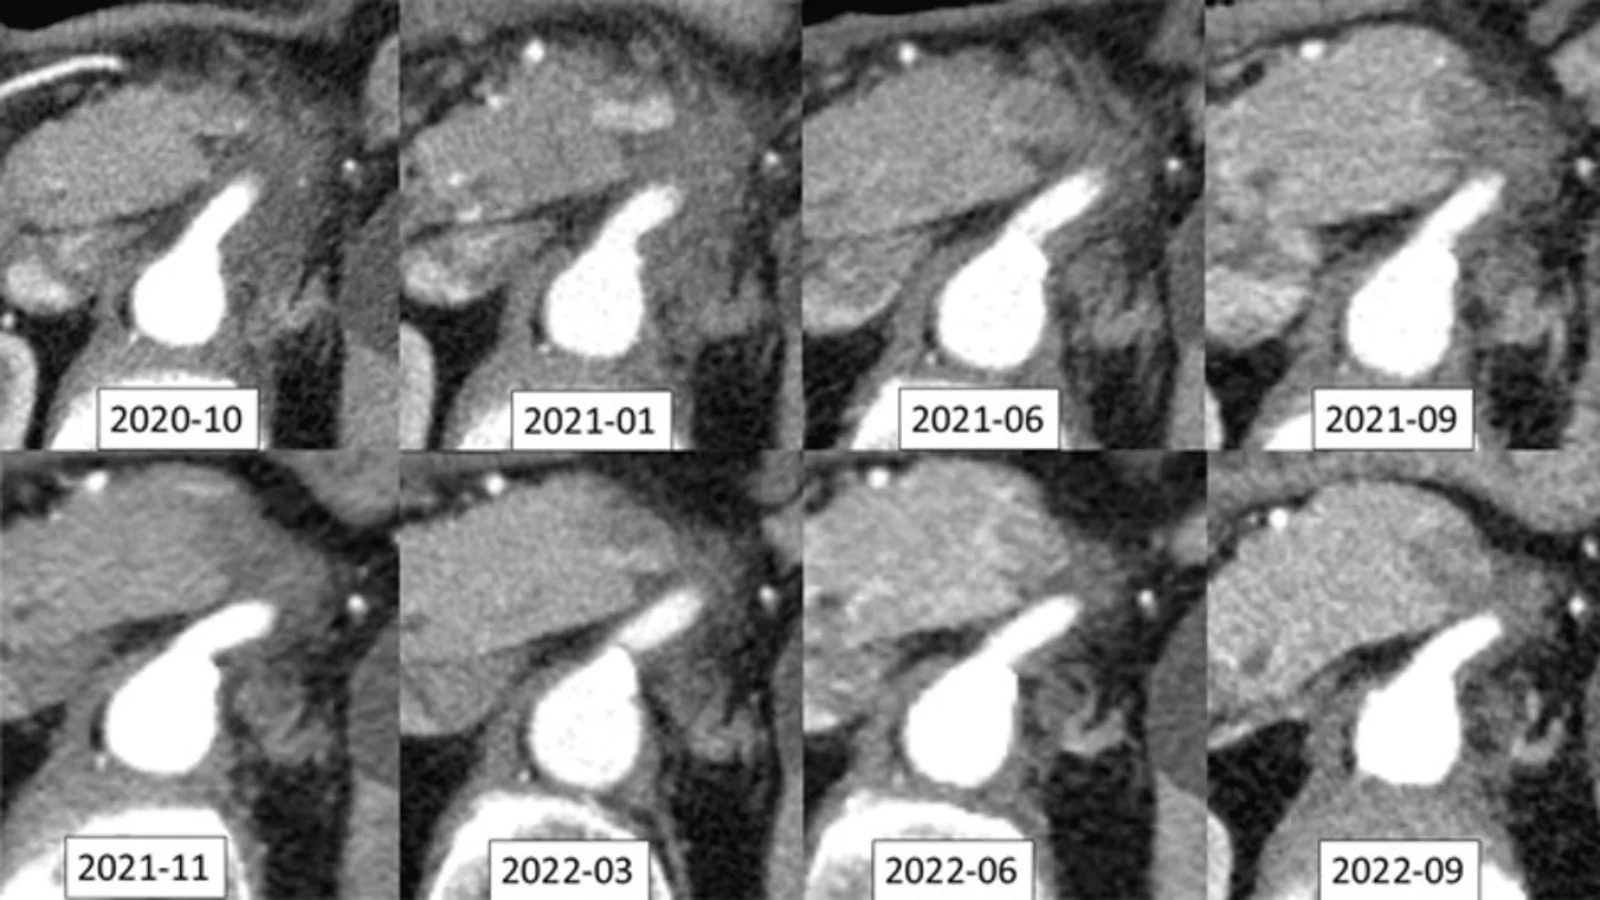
CT imaging of changes in primary tumor size and infiltration during liposomal irinotecan plus 5-FU treatment within proximity of the superior mesenteric artery 5-FU, fluorouracil; CT, computed tomography.
